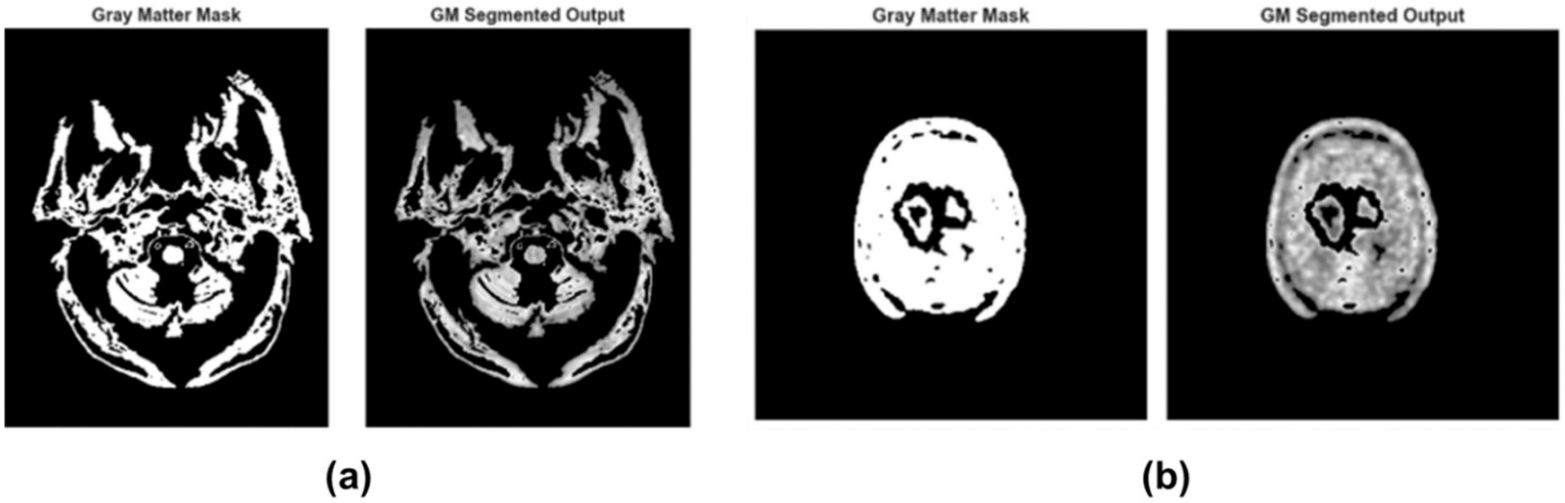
Feature extracted image of (a) PET and (b) MRI.
